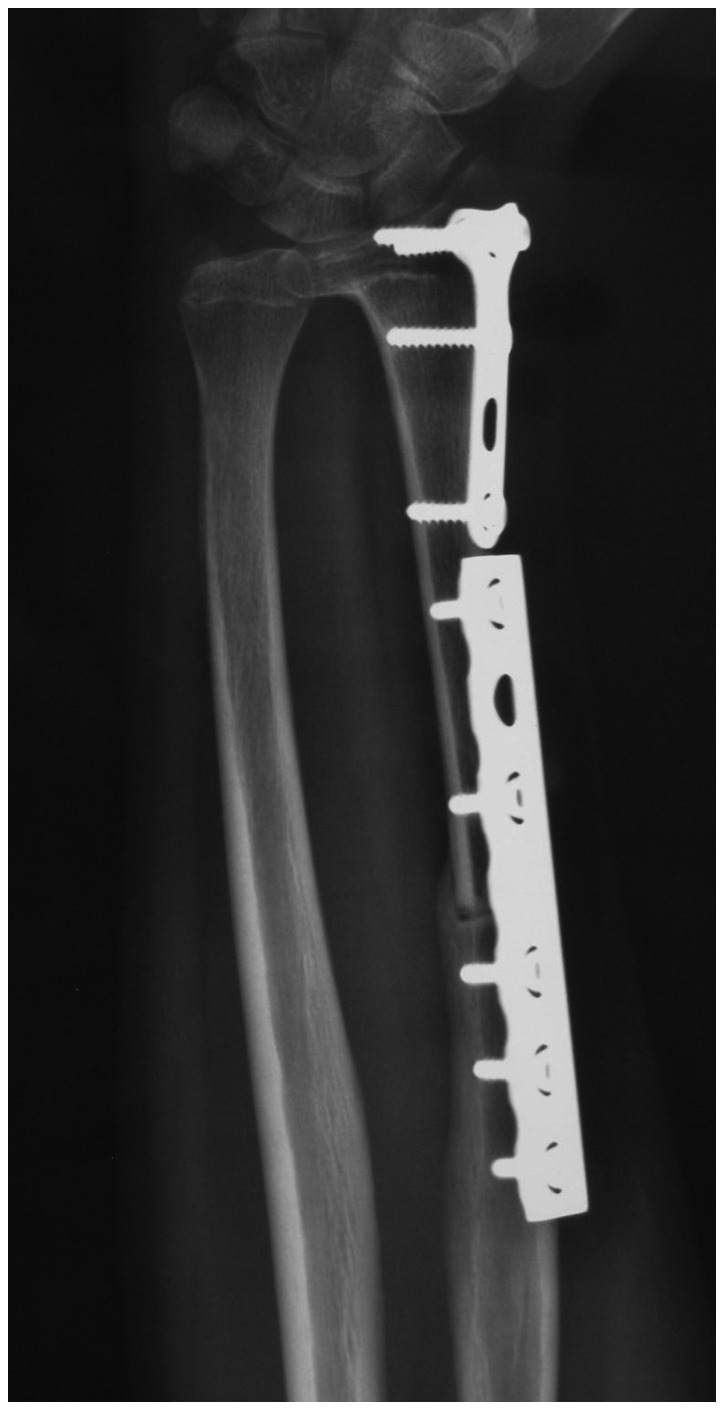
Six months after surgery, plain radiography revealed that the grafted fibular bone had healed well to the host bone.
